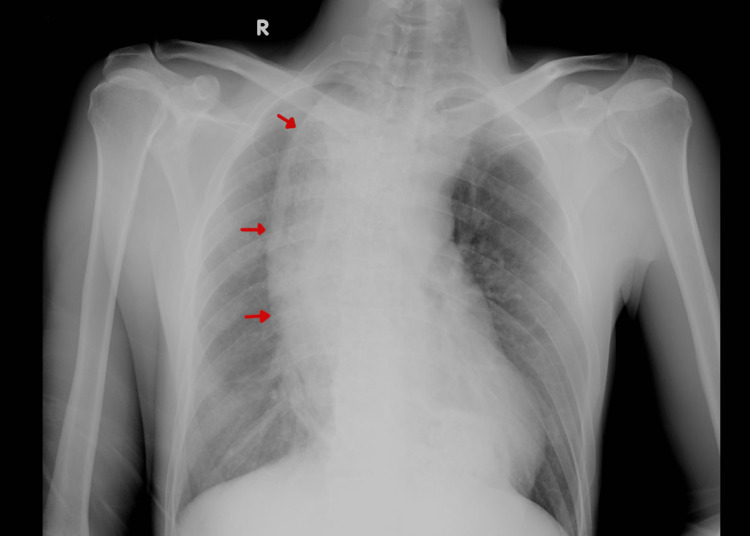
Chest X-ray (anteroposterior view) with red arrows pointing to the noticeably dilated esophagus, which is shown to be smoothly tapering below to the gastroesophageal junction.
